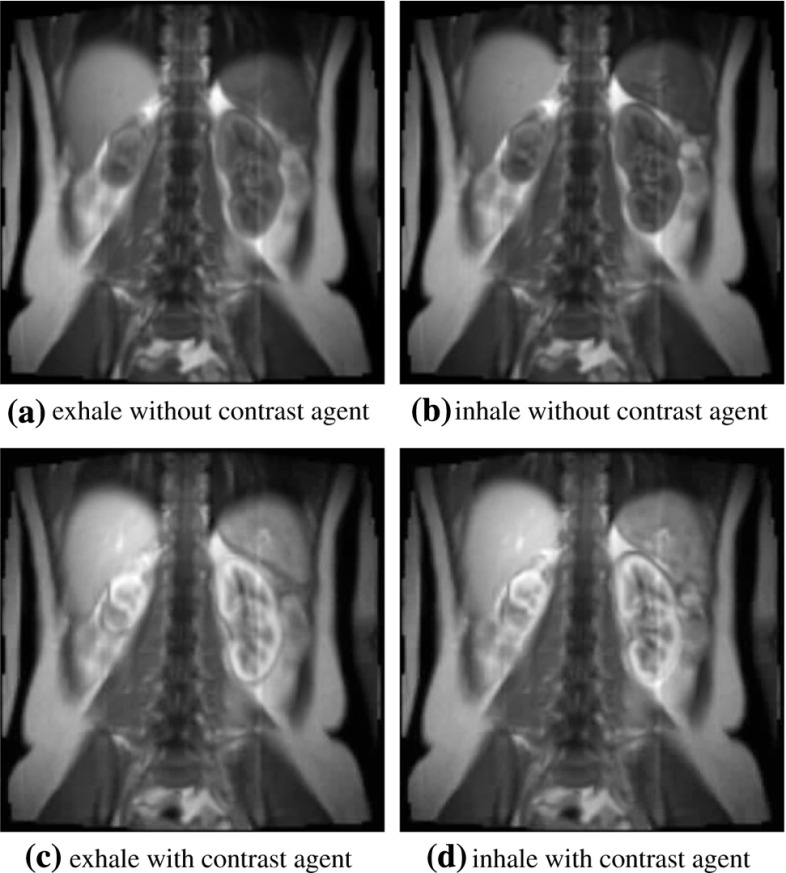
Representative stages of the DCE-MRI sequence: exhale and inhale with and without contrast agent, where these are defined by (9.1)
